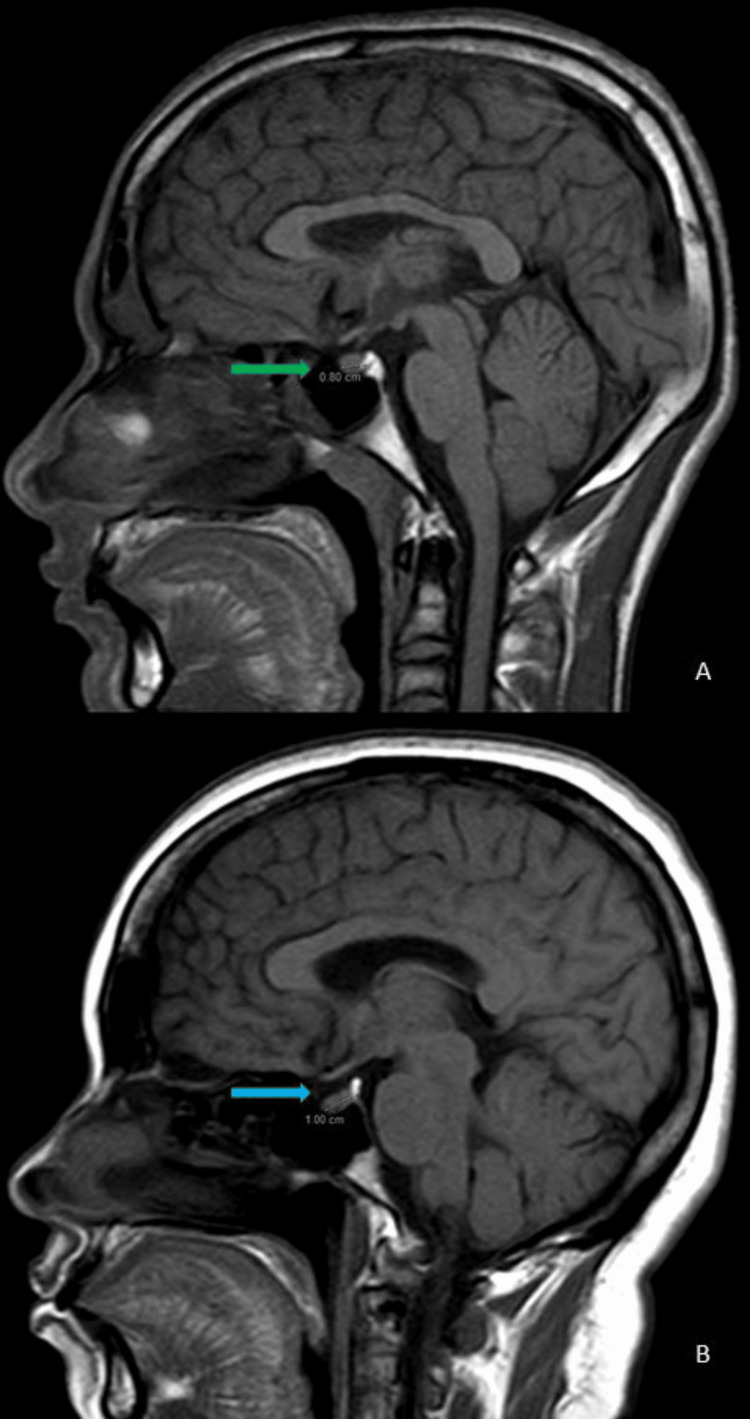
(A, B): MRI of the sella turcica. (A) T1 mid-sagittal: the pituitary gland in a 45-year-old female shows an anteroposterior dimension of 8 mm (green arrow). (B) T1 mid-sagittal: the pituitary gland in a 25-year-old male shows an anteroposterior dimension of 10 mm (blue arrow)
